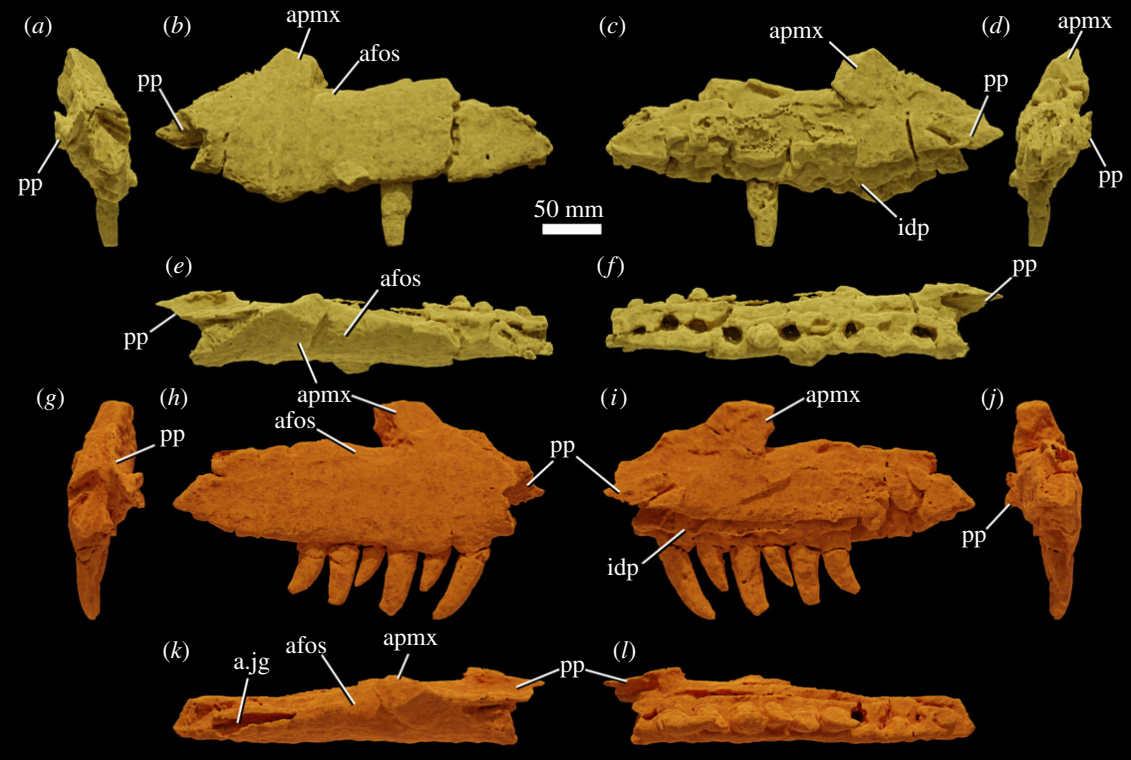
Maxillae of NHMUK R36620, holotype of *Mambawakale ruhuhu*, reconstructed from CT data. Left maxilla in anterior (*a*), lateral (*b*), medial (*c*), posterior (*d*), dorsal (*e*) and ventral (*f*) views. Right maxilla in anterior (*g*), lateral (*h*), medial (*i*), posterior (*j*), dorsal (*k*) and ventral (*l*) views. Abbreviations: afos, antorbital fossa; a.jg, articular surface for the jugal; apmx, ascending process of the maxilla; idp, interdental plates; pp, palatal process.
